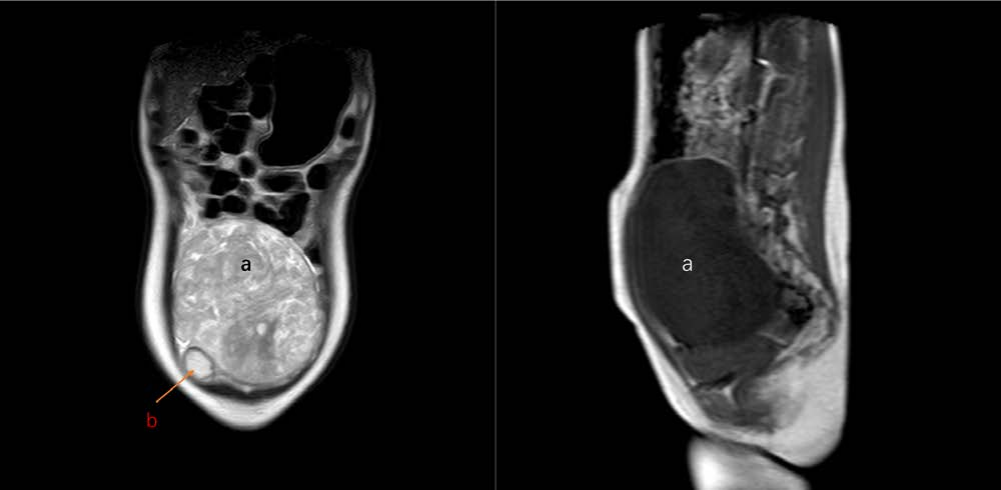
Abdominopelvic enhanced MRI revealed a large mass (a) with heterogeneous enhancement. The posterior margin of mass (a) is closely adjacent to the presacral region and causes significant compression of the sigmoid colon; its superior margin extends to the level of the fourth lumbar vertebra (L4), and inferior margin is closely adherent to the posterior uterine wall. Mass (b) is predominantly cystic, unilocular, and contains fatty components.
